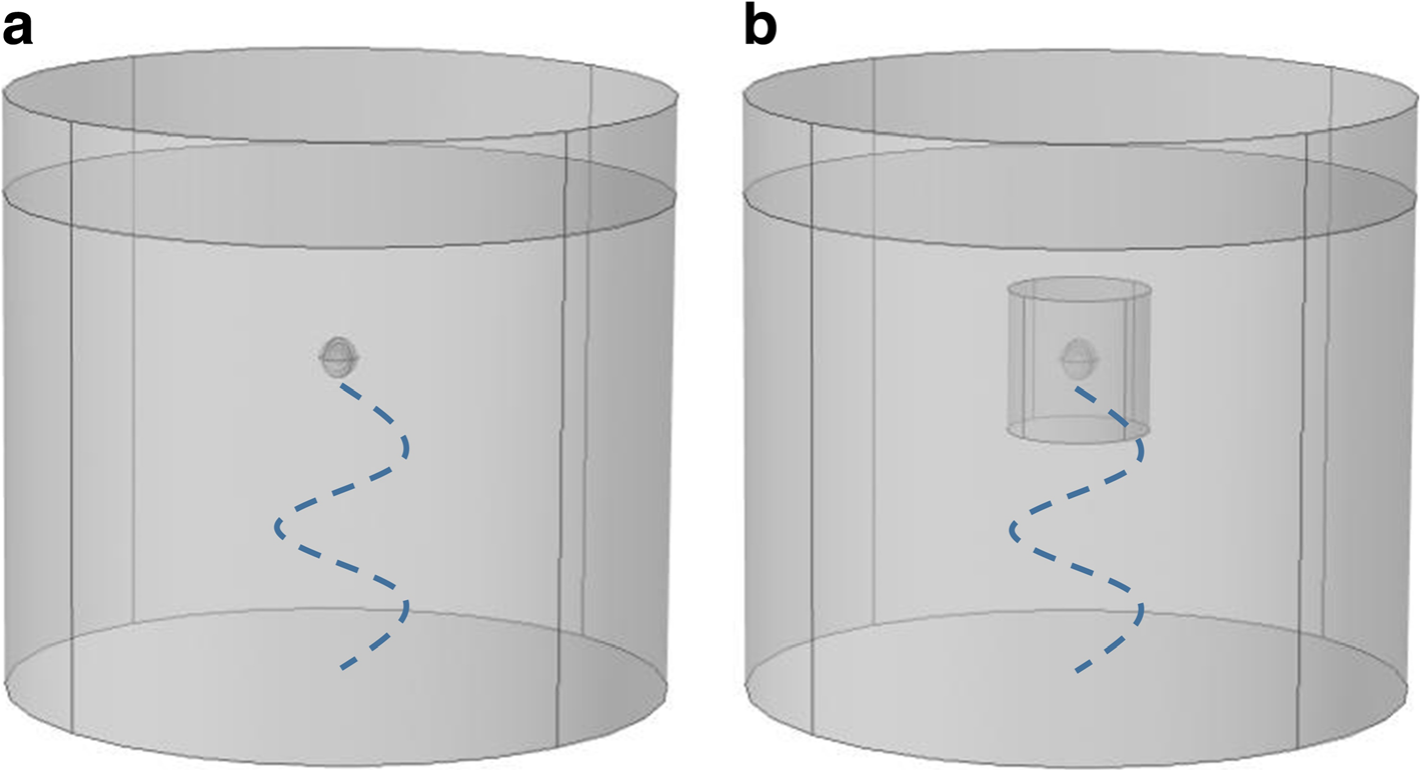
Model Geometry: a) A suspended cell (indicated by the small sphere) immersed in growth media. The ultrasound source (14 kPa) is positioned at the bottom and is indicated by the blue dotted line. The cell position is frequency dependent to ensure the position remains at an antinode. b A chondron (indicated by the sphere) embedded in the extracellular matrix (indicated by the cylinder) and immersed in growth media. The ultrasound source is positioned at the bottom (blue dotted line). The cell position is frequency dependent
